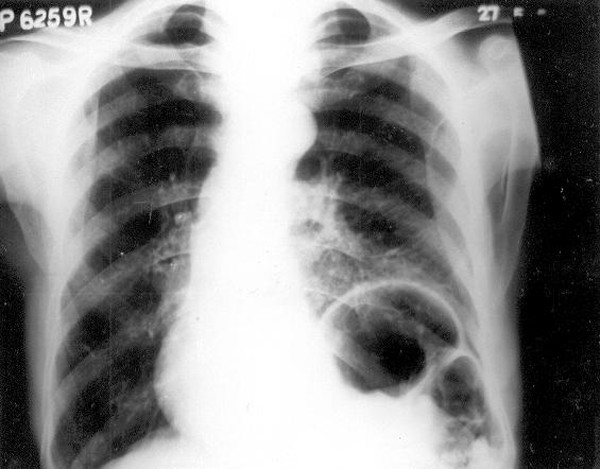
Chest roentgenogram revealing an elevated left hemidiaphragm and a shift of the lower mediastinum to right.
